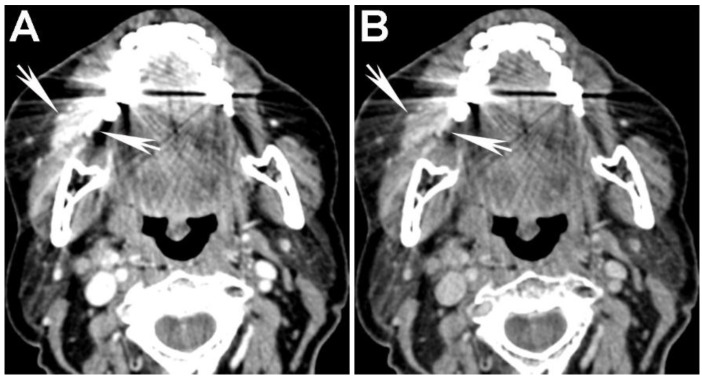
Use of high energy DECT virtual monochromatic images (VMIs) for dental artifact reduction in a patient with a gingival-buccal tumor. (A) 65 keV (equivalent to single energy CT) and (B) 95 keV VMIs are shown from the same level in the neck. Note reduction of artifact and improved visualization of the enhancing tumor on the 95 keV VMI compared to the 65 keV VMI.
